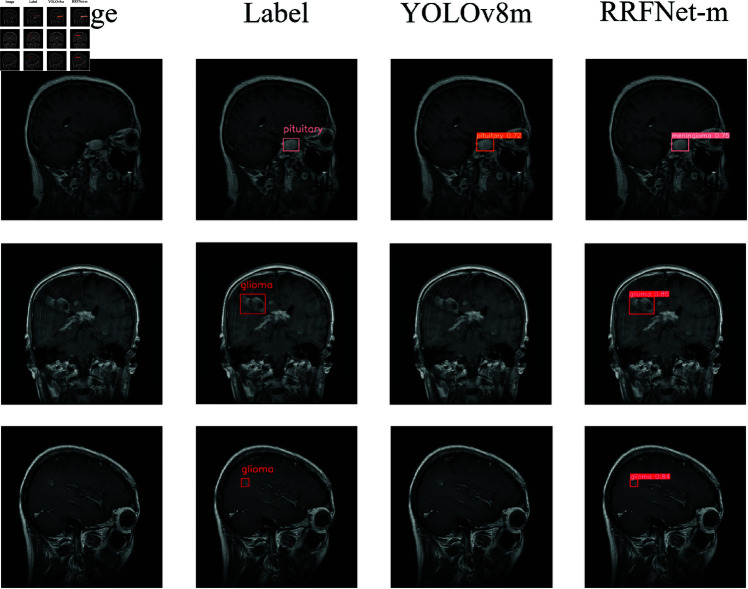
The brain tumor detection visualization comparison chart, from left to right, shows the brain tumor MRI image, the annotated brain tumor region label, and the detection visualization results of YOLOv8m and RRFNet-m.
